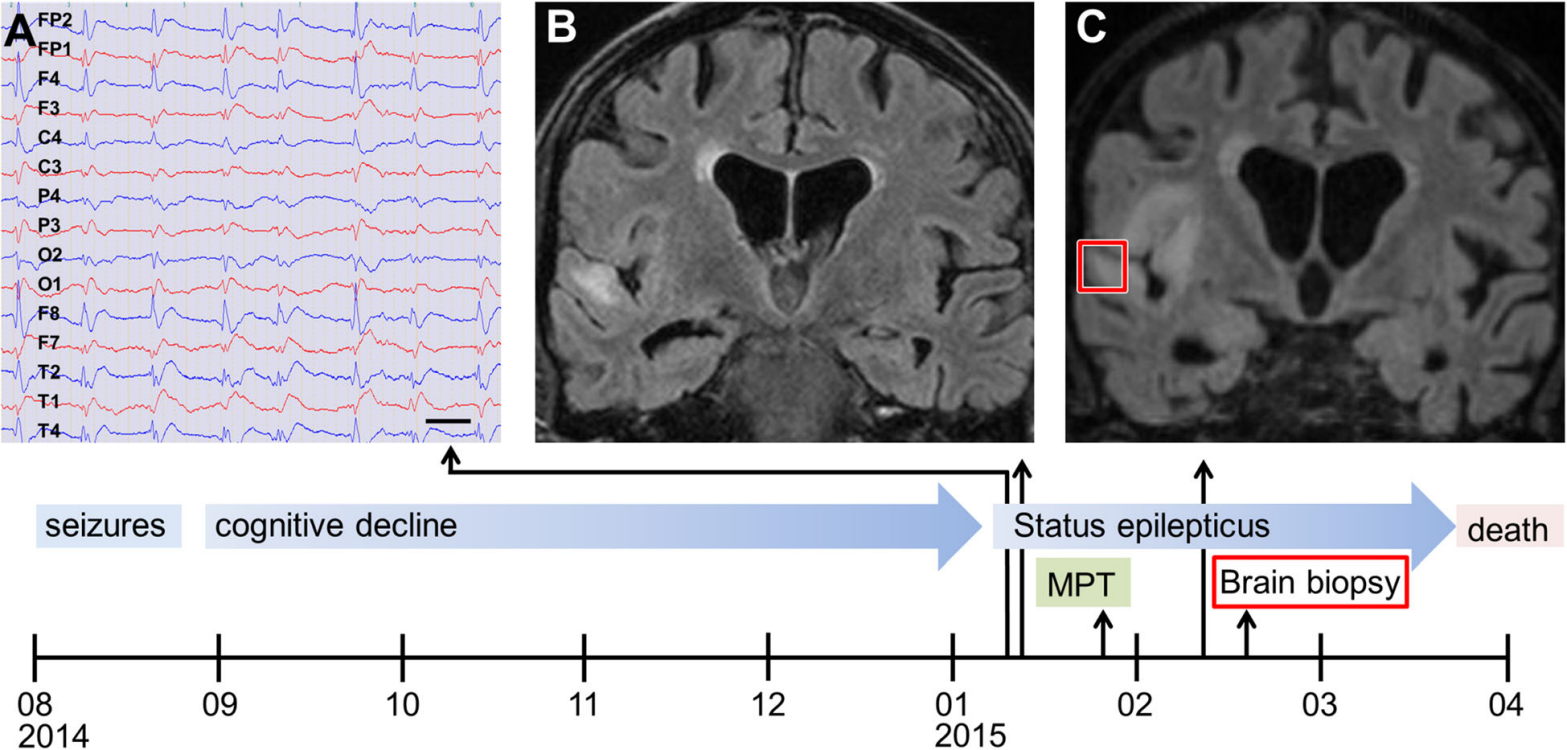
Clinical course, EEG and MRI of the index patient. Time axis of disease development with main symptoms, treatments and findings. a EEG excerpt in average potential reference montage shows right-sided periodic lateralized epileptiform discharges (PLED); bar: 1 s. b Coronal T2-FLAIR MRI on admission shows hyperintensity in the right superior temporal gyrus, compatible with inflammation. c Coronally reconstructed 3D-FLAIR MRI shows progression after 30 days. Abbreviations: MPT, methylprednisolone pulse therapy; T2-FLAIR, T2-weighted fluid attenuation inversion recovery; 3D-FLAIR, three dimensionally acquired fluid attenuation inversion recovery. Red square indicates site of brain biopsy
